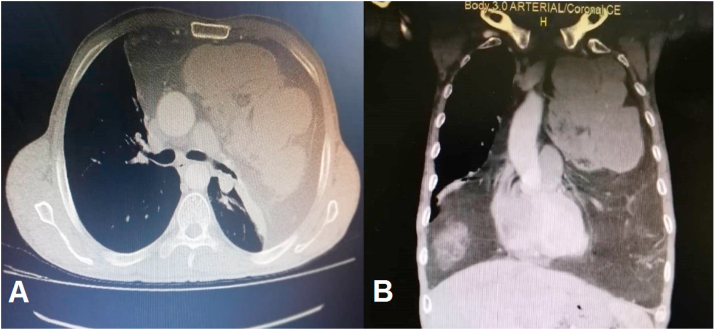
(a) Coronal and (b) sagittal Computed tomography demonstrated an anterior mediastinal tumor with irregular soft tissue density.
